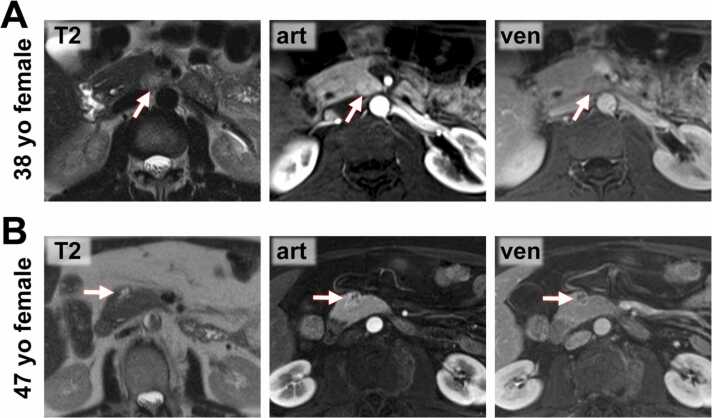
MR images from pNEN patients. A) 38-year-old female patient with a small pNEN in the medial part of the pancreatic head/ uncinated process (*arrow*). The lesion lacks a definite T2-hypointense capsule. It shows moderate arterial (art) hyperenhancement. B) 47-year-old female patient with a small pNEN in the pancreatic head with central cystic degeneration, lack of a definite T2-hypointense capsule, and marked enhancement in the arterial and venous (ven) phase (*arrow*).
